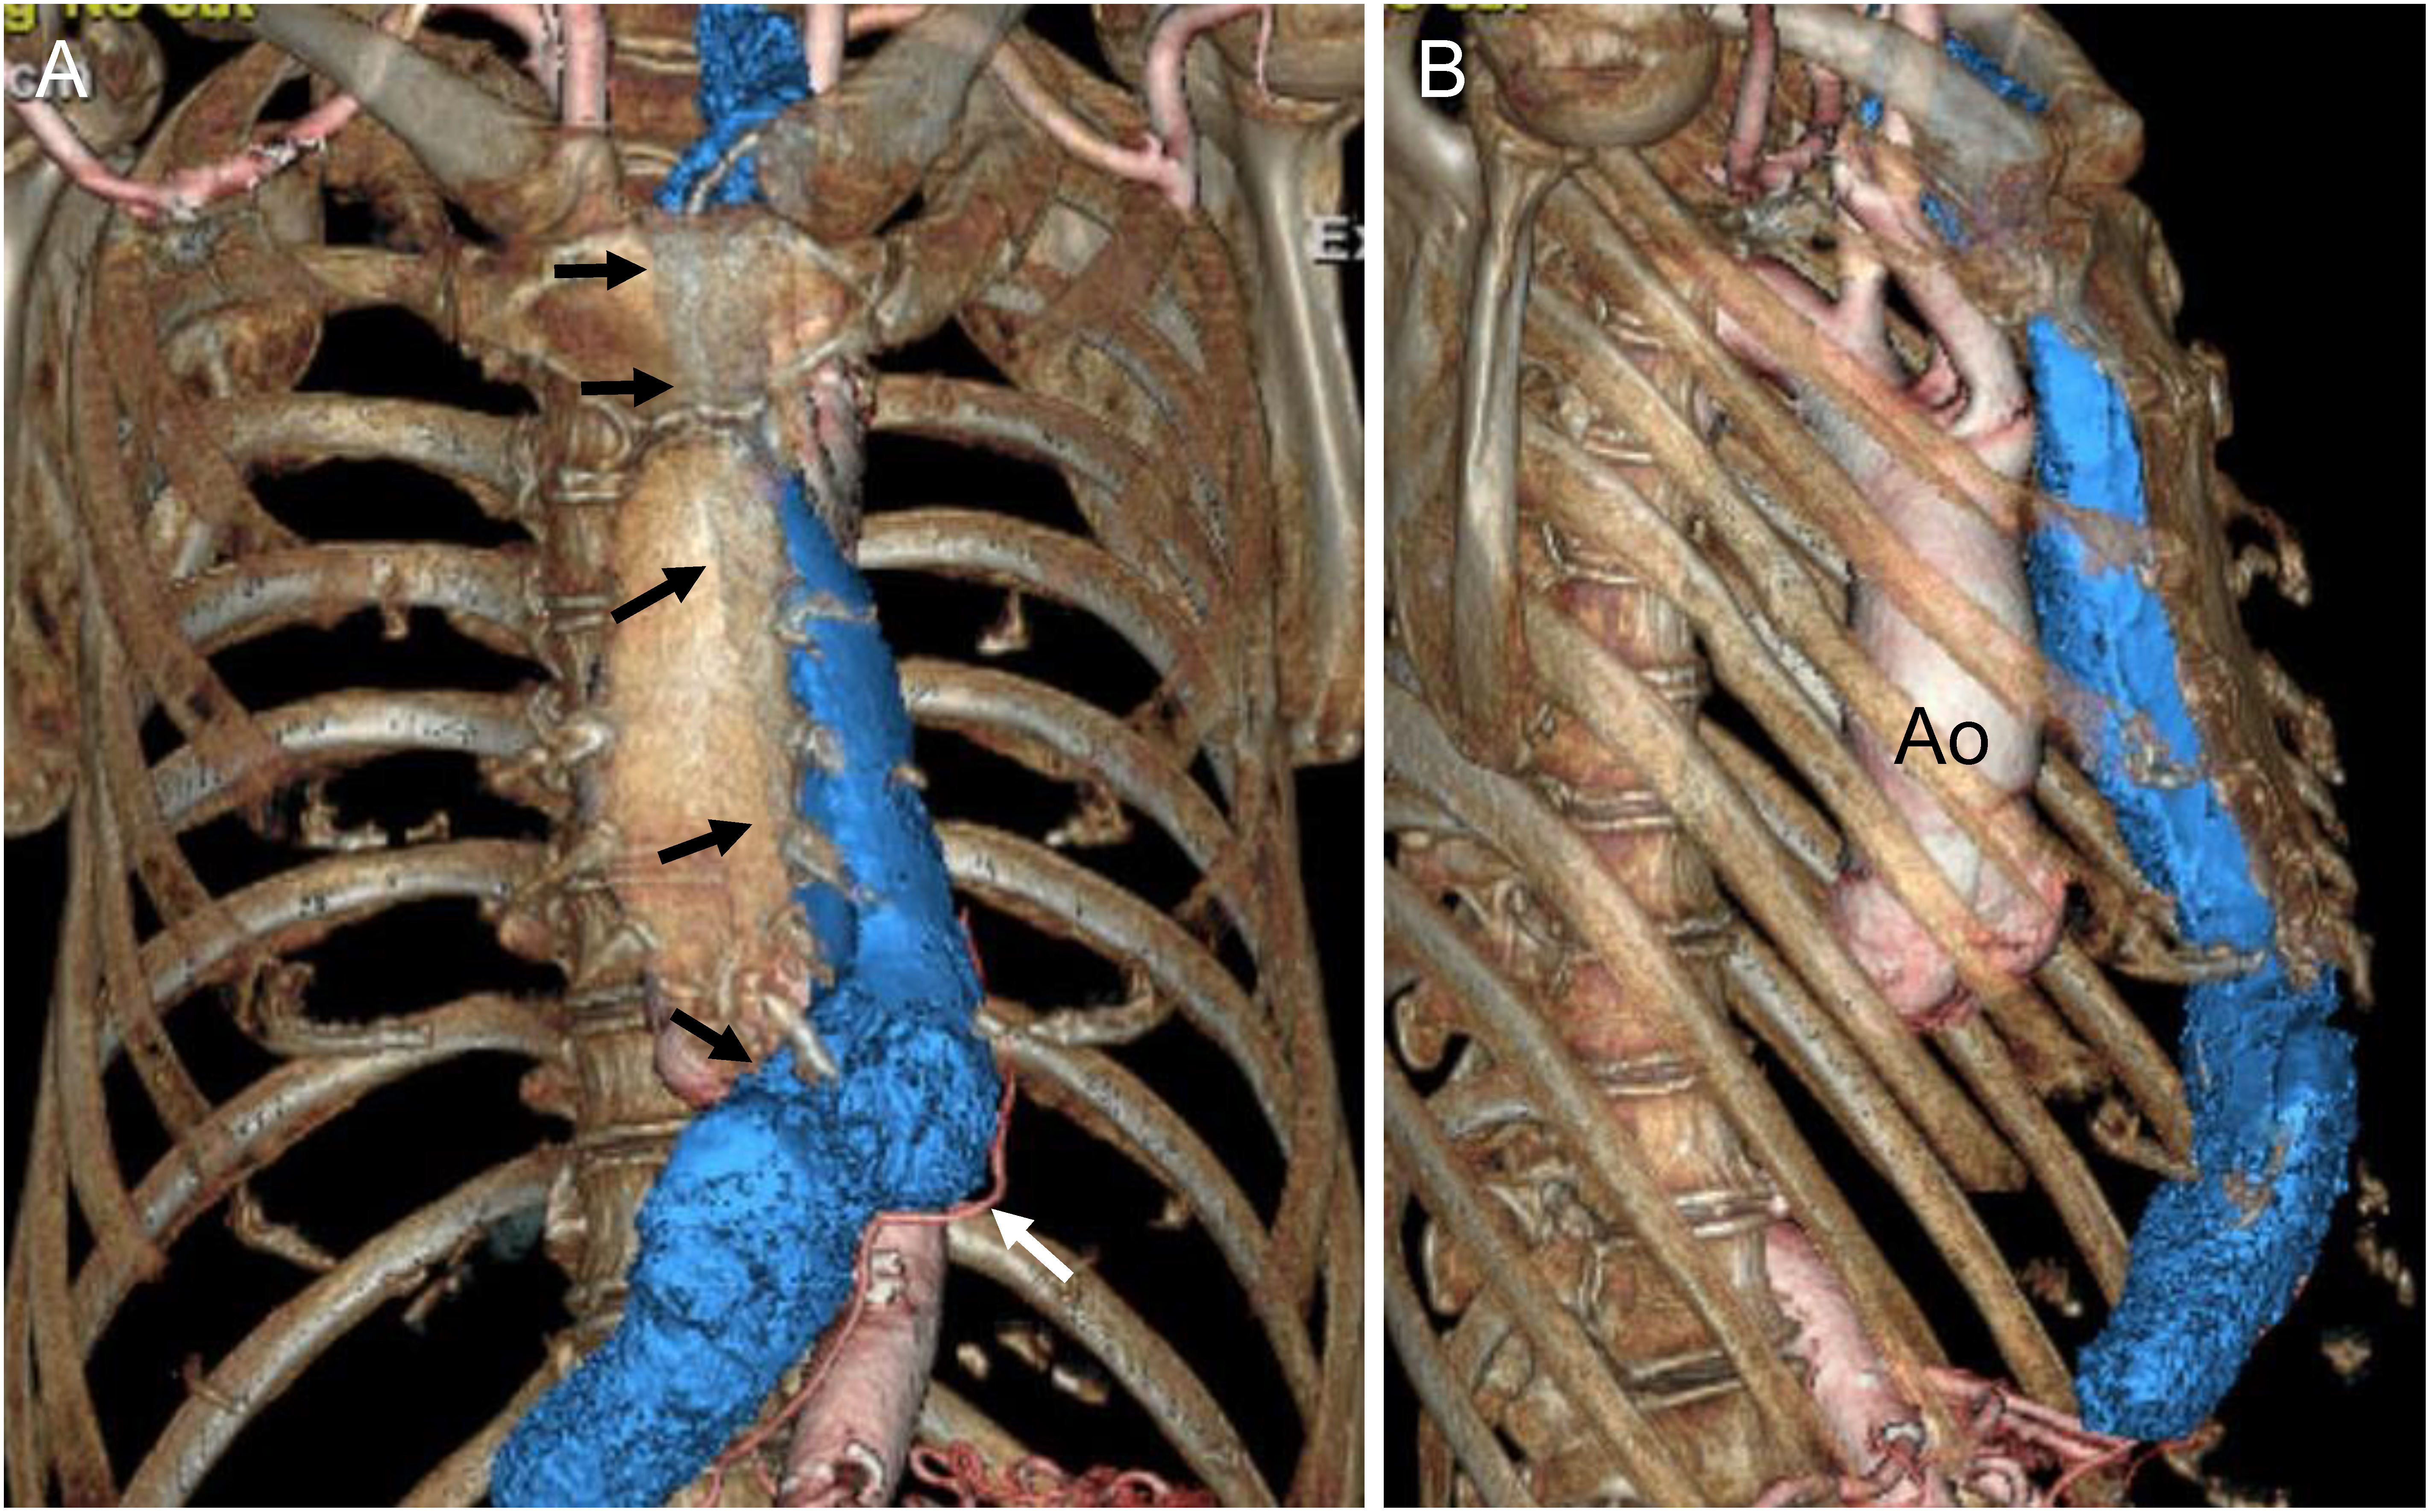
Fig. 1 Preoperative three-dimensional computed tomography. (A) Anterolateral view, (B) lateral view. The substernal gastric tube (blue) is on the midline behind the manubrium of the sternum and around the xiphoid process, but shifted to the left at the body of the sternum (black arrows). The right gastroepiploic artery located at the left side of the gastric tube (white arrow).
